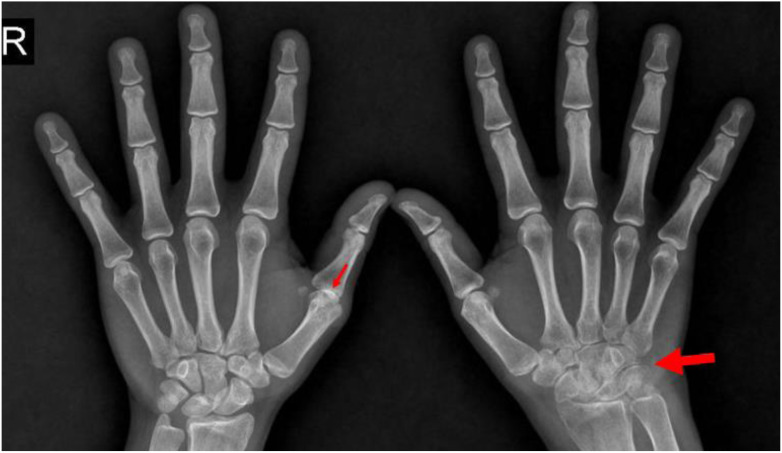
The hand x-ray of the above patient: Peripheral joint ankylosis in the first interphalangeal joint of the right hand, and joint space narrowing in multiple carpometacarpal, intercarpal, and radiocarpal joints of the left hand.
